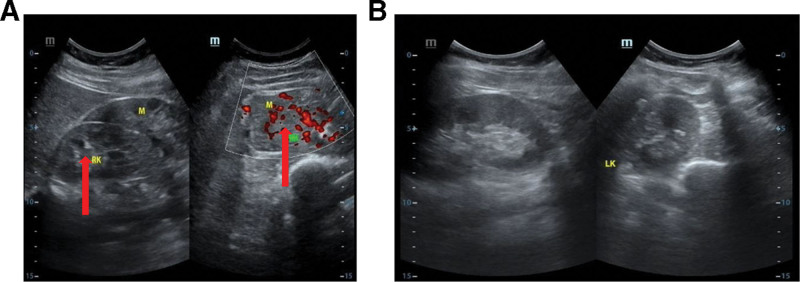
Preoperative ultrasonographic characterization of a large right retroperitoneal mass.
